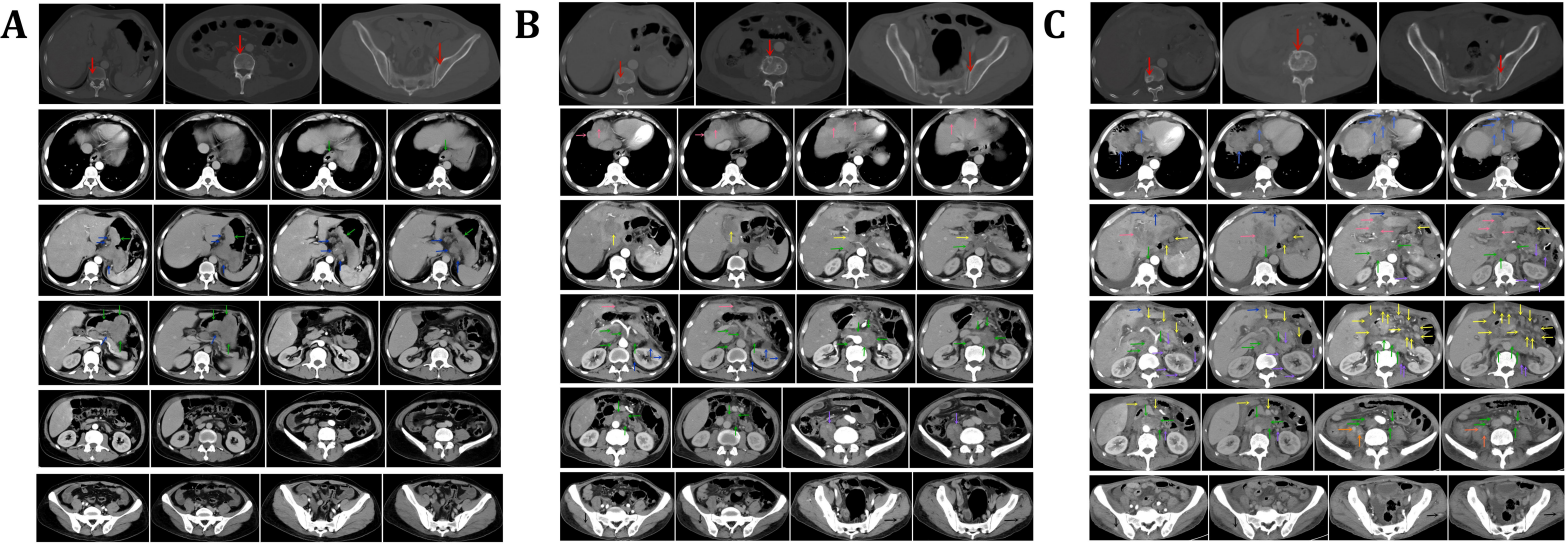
Abdominal CT imaging findings. (A) Contrast-enhanced abdominal CT on June 6, 2021, prior to surgery, revealed bone metastases (red arrow), gastric cancer (green arrow), and confluent perigastric lymphadenopathy (blue arrow). (B) After two cycles of chemotherapy, contrast-enhanced CT on August 28, 2021, showed bone metastases (red arrow); liver metastases and perihepatic lymphadenopathy (pink arrow); confluent lymph nodes at the hepatic hilum and fissure (yellow arrow); retroperitoneal (green arrow) and perirenal lymphadenopathy (blue arrow); metastases in the psoas major muscle (purple arrow) and gluteal intermuscular space (black arrow). (C) After four chemotherapy cycles, contrast-enhanced CT on October 13, 2021, revealed bone metastases (red arrow); liver metastases with perihepatic and hepatic fissure lymphadenopathy (blue arrow); intra-abdominal confluent lymphadenopathy (yellow arrow); retroperitoneal (green arrow) and perirenal (purple arrow) lymphadenopathy; as well as metastases in the psoas major (orange arrow) and gluteal intermuscular region (black arrow).
